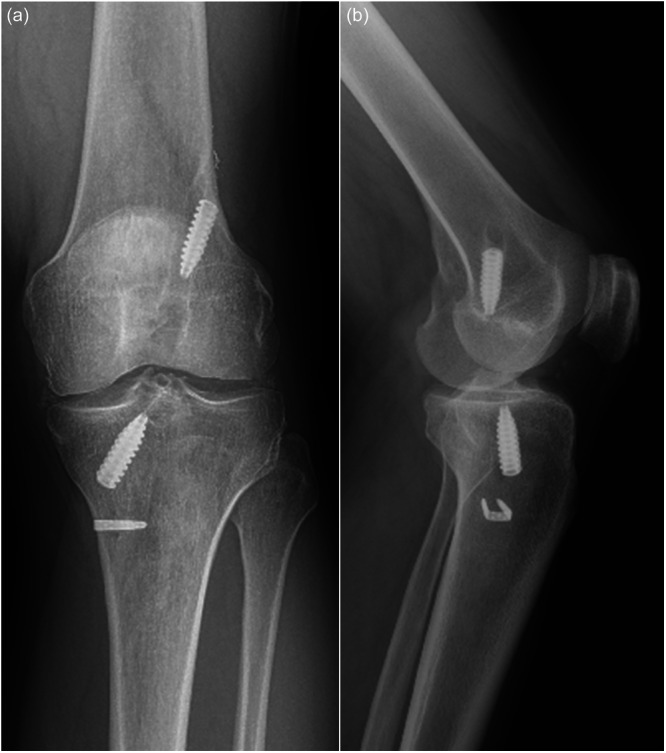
An anteroposterior (a) standing weight‐bearing radiograph and a lateral (b) radiograph of the left knee, obtained 6 years after ligament advanced reinforcement system (LARS) device implantation, revealed Grade III arthritic changes.
